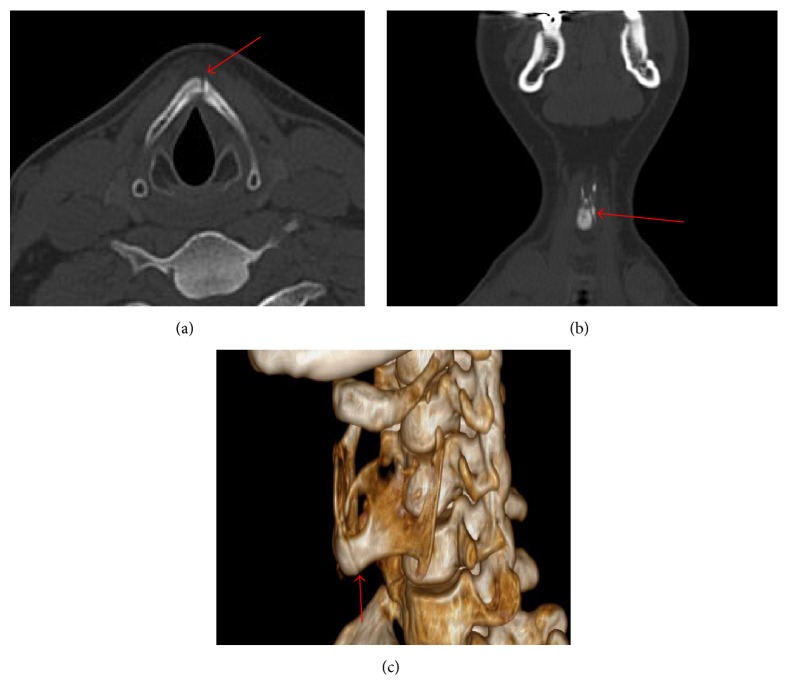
Computed tomography, bone window. Left, complete, and nondisplaced parasagittal fracture that compromises thyroid cartilage (red arrows). (a) Axial view; (b) coronal view; (c) 3D bone reconstruction.
